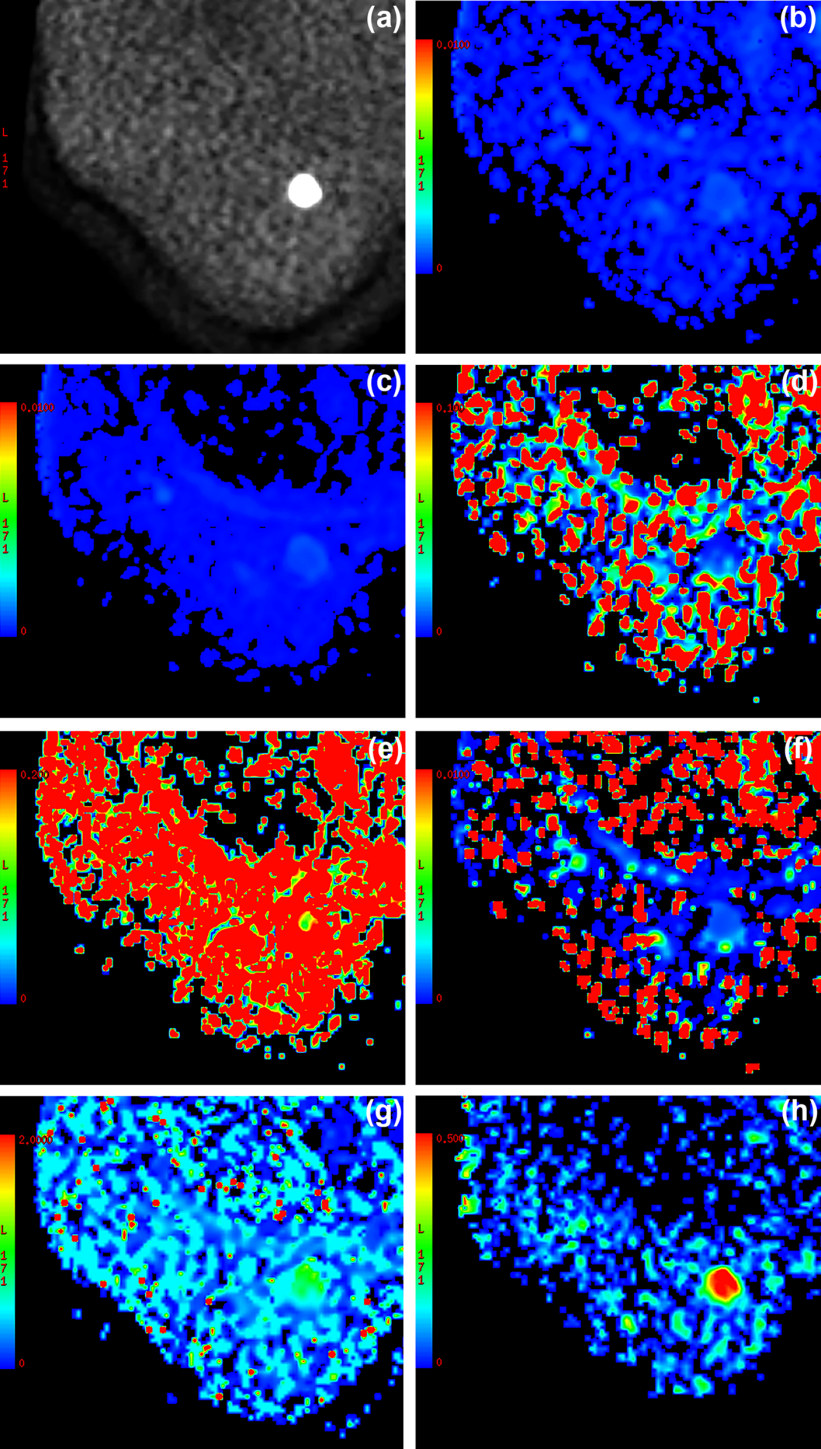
A 61-year-old patient was pathologically confirmed with malignant breast invasive ductal carcinoma on the left breast correlating area, measured 33 mm $$\times$$ 26 mm. DWI image with b-value of 1000 s/mm^2^ (a) shows the ROI on the whole tumor and displays the ROI at tumor margin. Parametric maps of (b) average standard ADC: 0.68 × 10^−3^mm^2^/s; (c)
